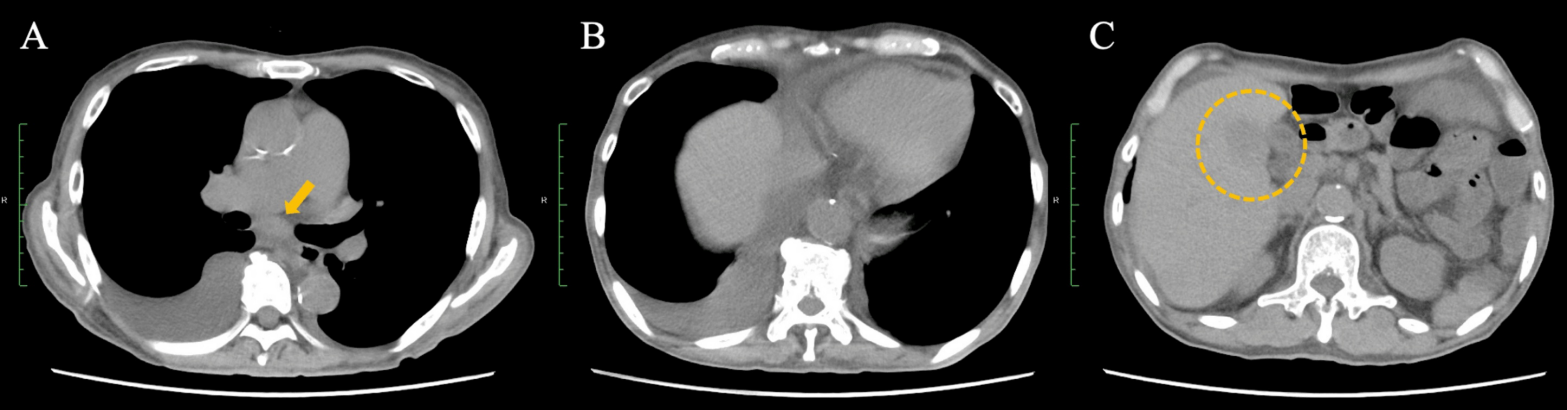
CT images obtained after two cycles of treatment with nivolumab plus ipilimumab (A, B) Chest CT shows a marked decrease in the right pleural effusion with a concurrent reduction in mediastinal lymph node size; pleural dissemination is no longer visualized (arrow). (C) Abdominal CT likewise demonstrates shrinkage of the intrahepatic metastatic lesions (dotted circle). The response to nivolumab plus ipilimumab was assessed as a partial response according to Response Evaluation Criteria in Solid Tumors (RECIST) version 1.1 [7].
